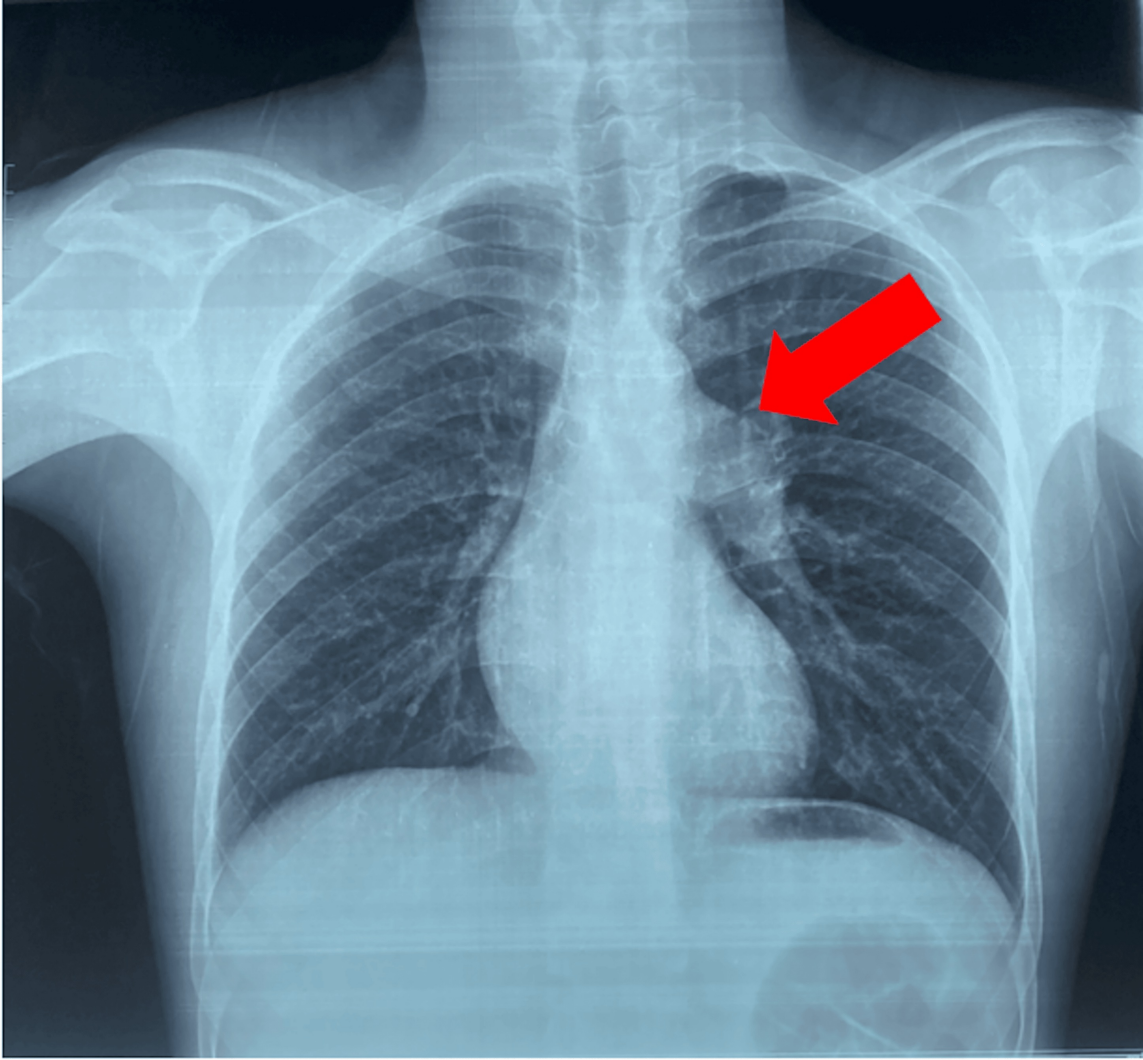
Posteroanterior Chest Radiograph Showing Left Pulmonary Artery Dilatation A prominent left pulmonary artery segment (red arrow) is seen, consistent with post-stenotic pulmonary artery dilatation in the setting of severe pulmonary valve stenosis. No signs of pulmonary congestion are observed.
